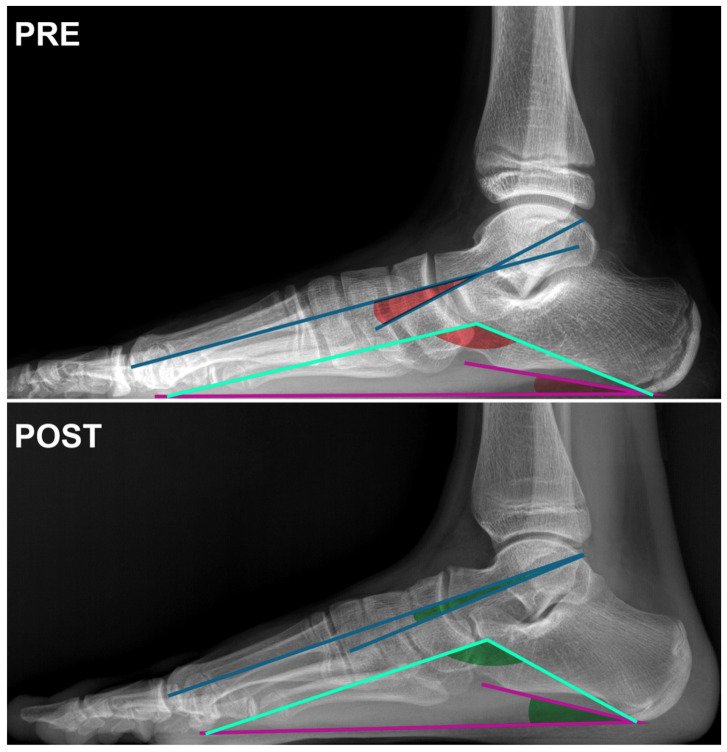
Lateral X-ray view of the foot of an 11-year-old female patient at the time of surgery (PRE) and at the 6-month postoperative follow-up (POST). Angle subtended by the blue lines: Meary’s angle; angle subtended by the cyan lines: Moreau-Bartani’s angle; angle subtended by the purple lines: Calcaneal Pitch angle.
